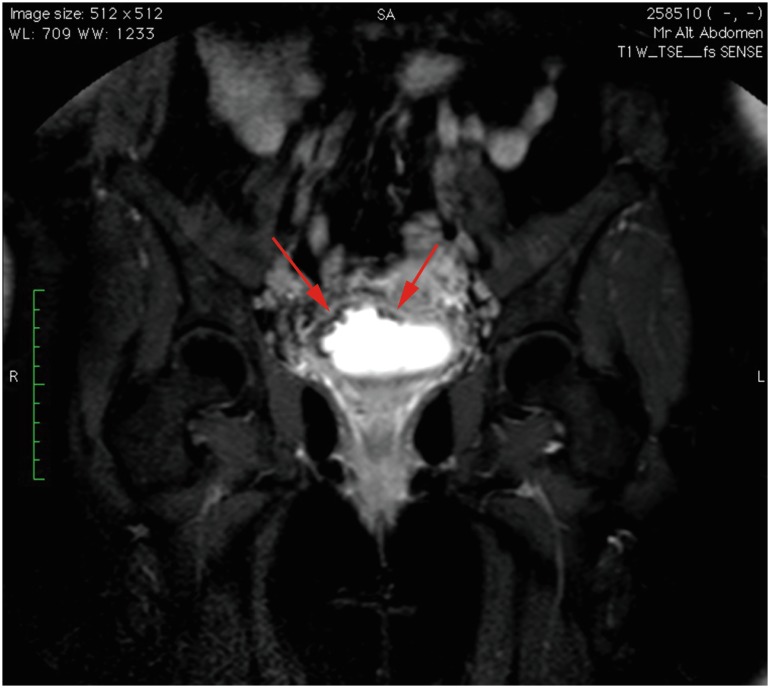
Coronal magnetic resonance image of bladder demonstrating filling defects (arrows) of right bladder wall.
